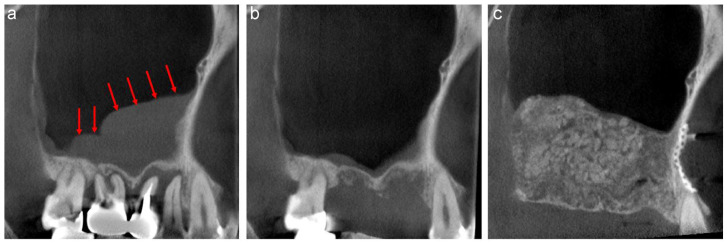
The mesiodistal section of CT images of the right maxillary sinus. (a) Antral, well-defined, faintly radiopaque lesions (red arrows) and marked alveolar bone resorption are observed near 16, 15, and 14. (b) The well-defined, faintly radiopaque antral lesions disappeared 3 months after extraction. (c) A radiopaque area is observed at the sinus floor elevation without swelling of the sinus membrane six months postoperatively.
